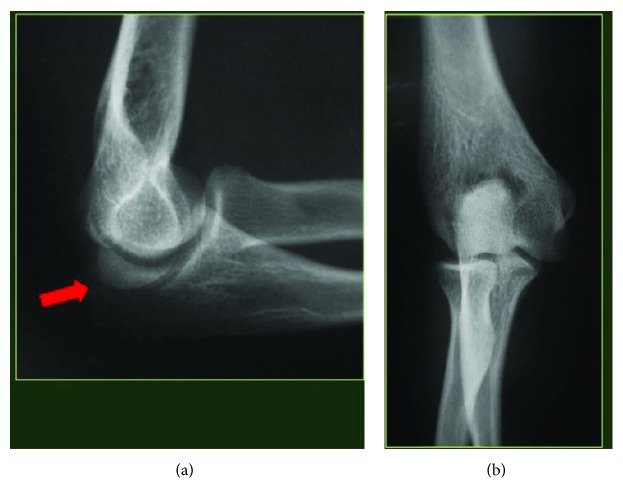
Case 2. (a, b) L and AP preoperative X-rays.
